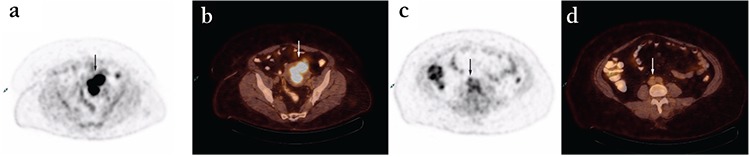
A 60-year-old female with endometrial cancer. Fluoro-D-glucose/positron emission tomography-computed tomography images demonstrate increased fluoro-D-glucose uptake in the tumor (a,b) and metastatic inter aortocaval lymph node (c,d)
